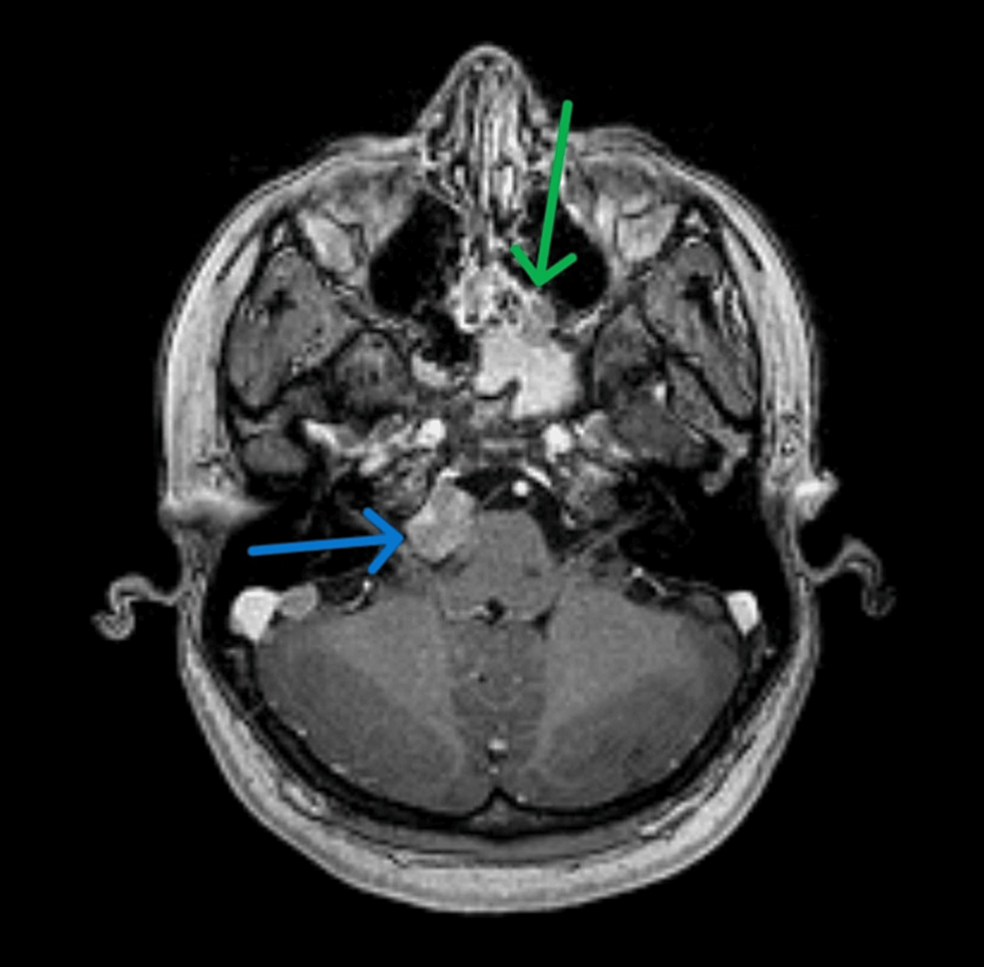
Axial T1 MRI with contrast. The green arrow indicates the lesion in the posterior ethmoidal cells. The blue arrow indicates a lesion in the right cerebellopontine angle causing slight compression of the pons.
